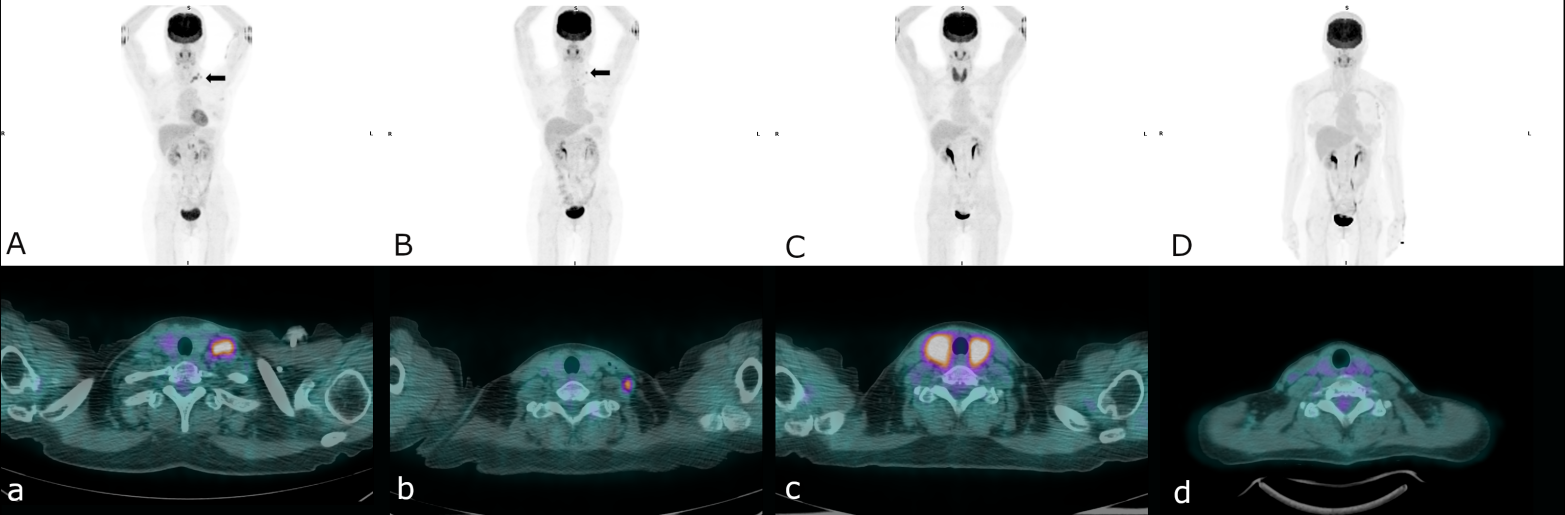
A serial pelvic MRI: T2-weighted sagittal and DWI-axial views and PET/CT images during treatment and follow-up (A) First recurrence at left supraclavicular lymph nodes (arrow), (B) Second recurrence at left lower neck lymph nodes (arrow), (C) Complete remission of second recurrence, thyroiditis was also noted, (D) Sustained free of disease since stopping immunotherapy.
